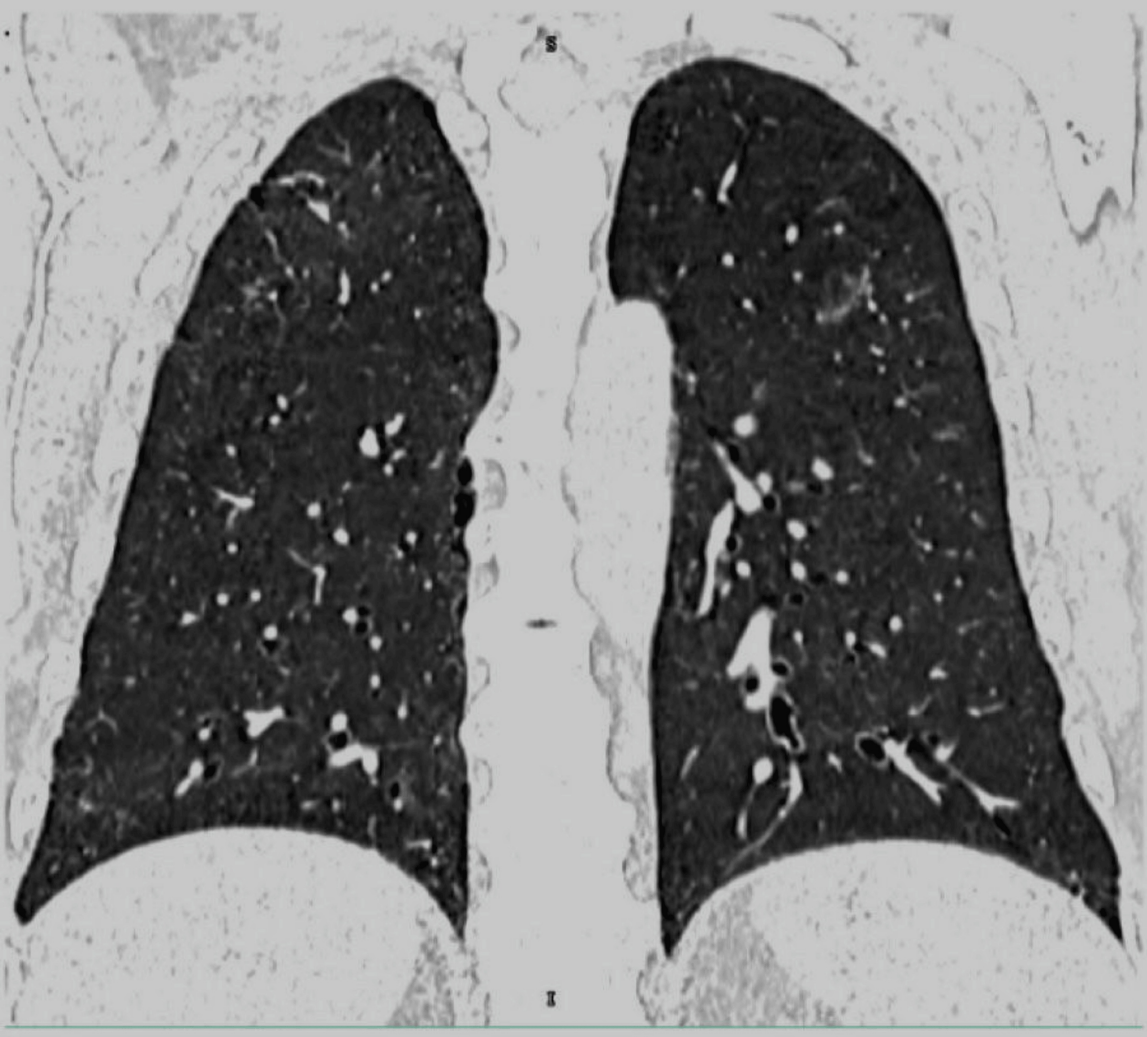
Coronal CT scan of the thorax showing diffuse solid micronodules in both lungs.
